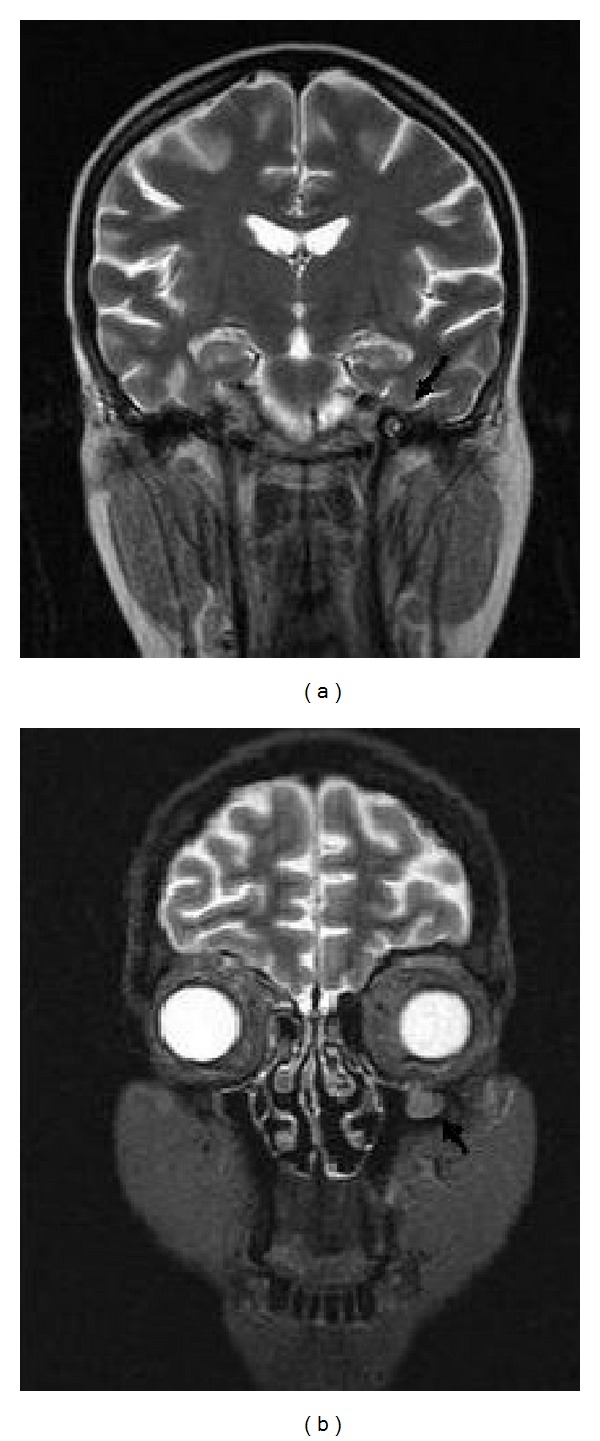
The cranial and orbital sagittal T2-weighted magnetic resonance image revealing a thickening of the third division of trigeminal nerve (a) and a soft tissue development of one centimeter diameter in the roof of left maxillary sinus (b).
